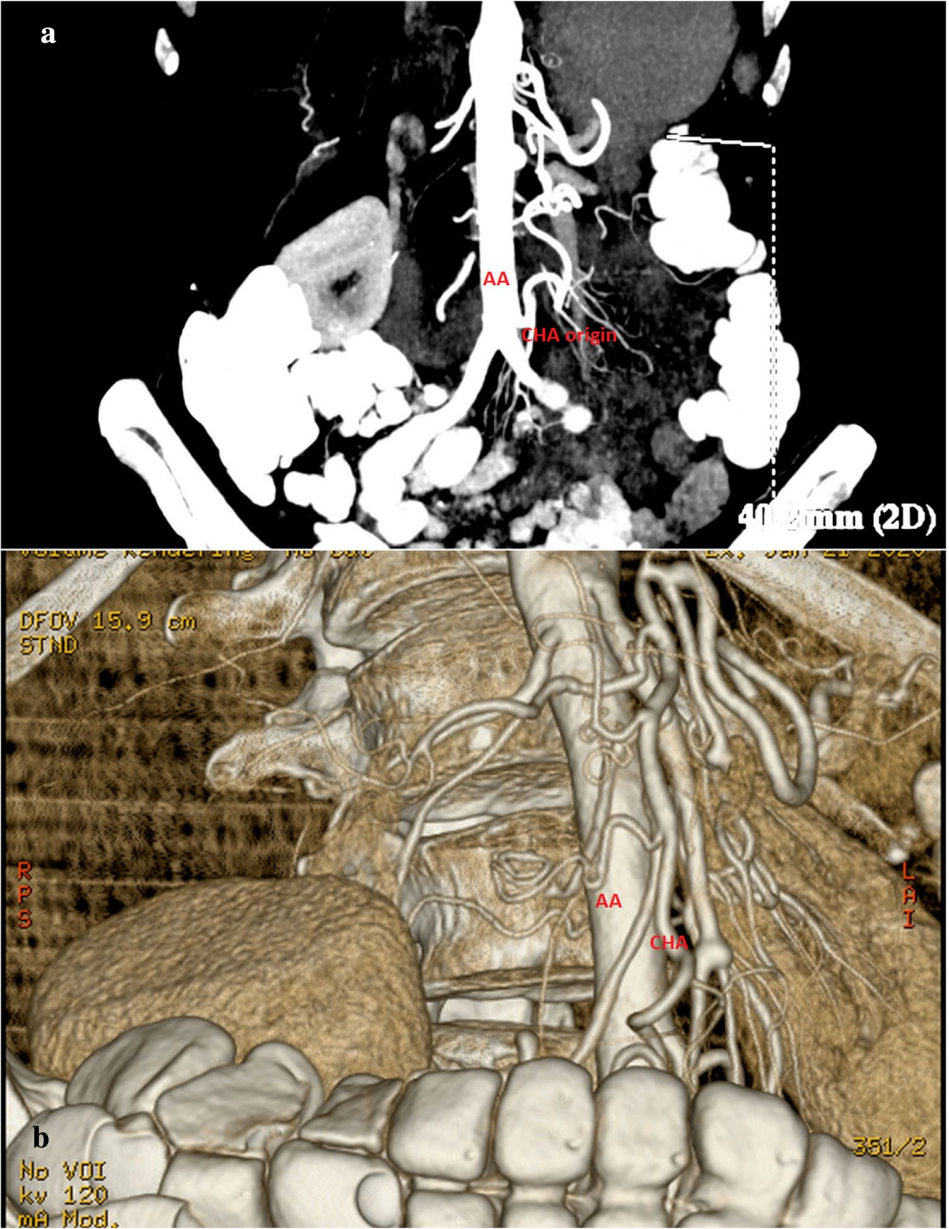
Computed tomography angiography (a) with reconstruction (b). An absence of CT. The left gastric (LGA), splenic (SA), and common hepatic (CHA) arteries connected above origin of the superior mesenteric artery (SMA) from the AA. SA and CHA filled with collateral circulation from the superior and inferior mesenteric arteries—a network of small vessels in the retroperitoneal space and at the head of the pancreas was visible. CHA arose directly from the anterior wall of abdominal aorta (AA) just above aortic bifurcation. The right hepatic artery (RHA) arose from the proximal CHA
